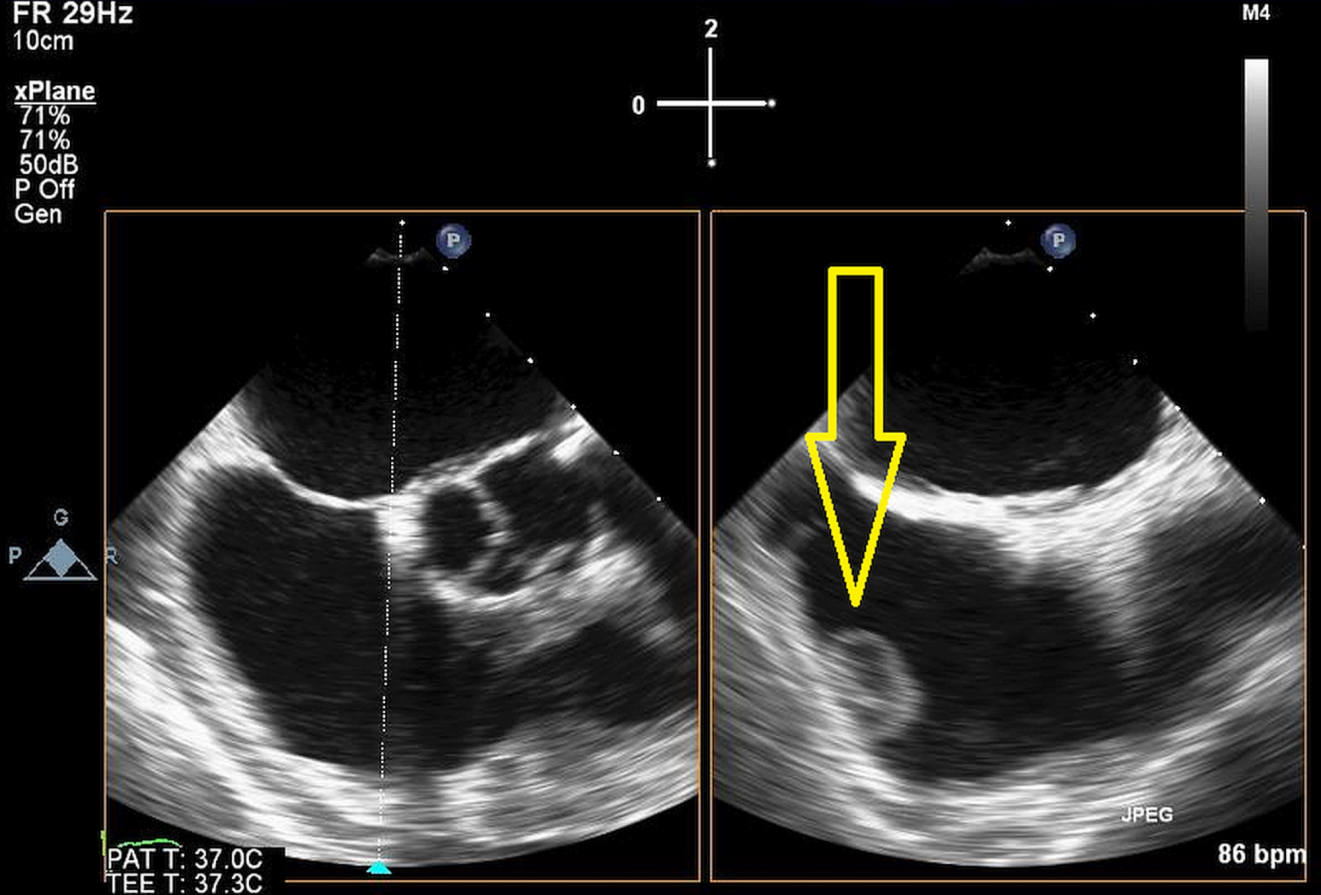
Transesophageal echocardiogram additional image. Arrow as shown displays 2D image of right heart mass and attachment onto the surrounding wall.
